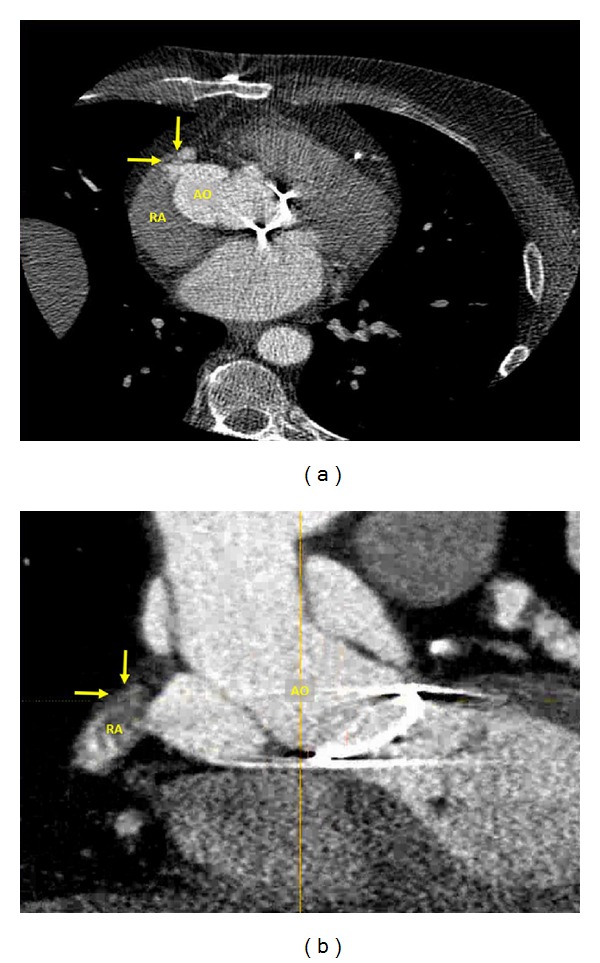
Cardiac CT scan demonstrates a contrast shunt within the wrapped portion of aorta with the right atrium during ventricular systole.
